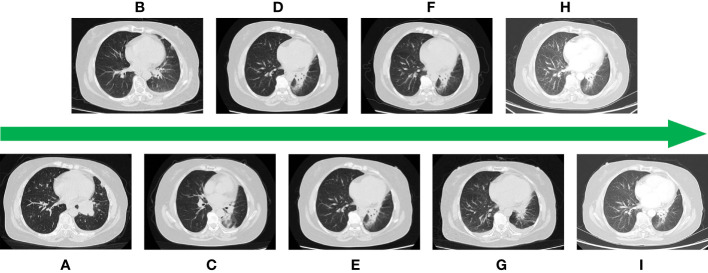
(A) CT image at the time of initial diagnosis. (B) Image after first-line treatment. (C) Image after second-line treatment. (D) Image after third-line treatment. (E) Image after fourth-line treatment. (F) Image after fifth-line treatment. (G) Image after sixth-line treatment. (H) Image after seventh-line treatment. (I) Image after eighth-line treatment.
